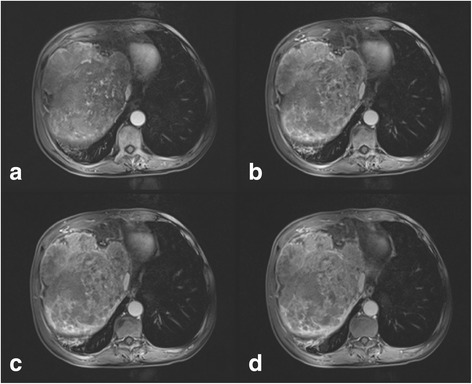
Transverse DCE-MRI of the abdomen shows a large lesion in the right lobe of the liver in four different phases. DCE-MRI showed heterogeneity of signal enhancement in the arterial phase (a), a more obvious heterogeneous signal enhancement in the venous phase (b), slightly weakened heterogeneous enhancement in the equilibrium phase (c), and a significantly weakened heterogeneous enhancement in the delayed phase (d)
